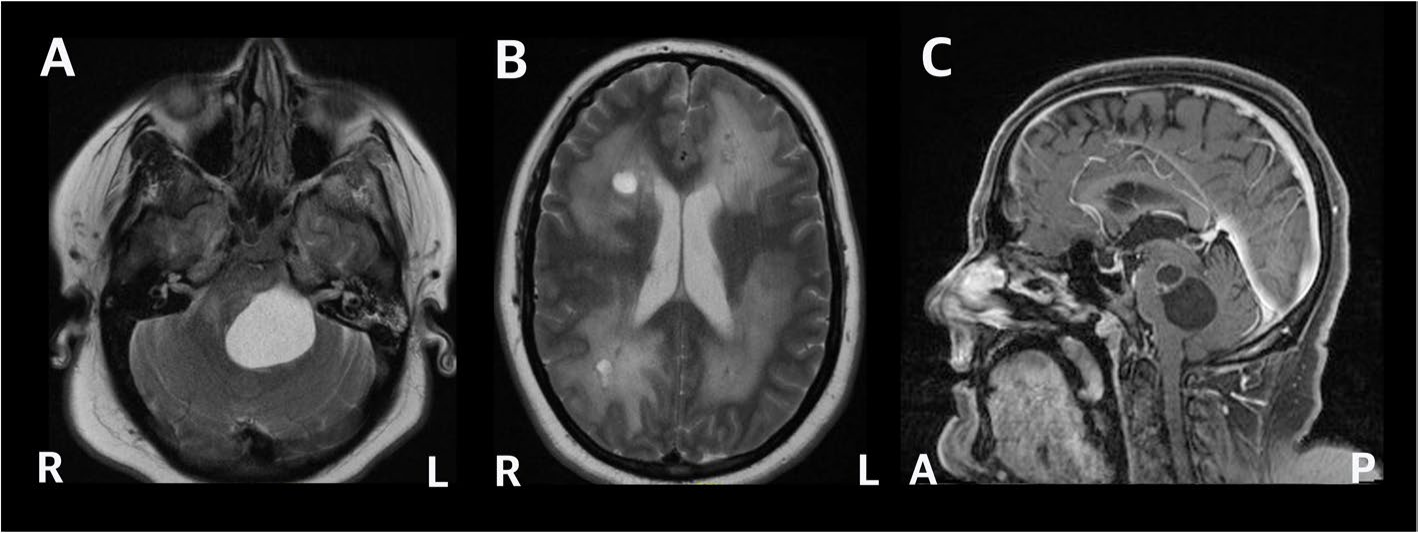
Magnetic resonance imaging (MRI) demonstrating LCC intracranial pathology. T2-weighted axial images demonstrated a cystic lesion in the posterior fossa exerting mass effect upon the brainstem (A) and diffuse T2-hyperintense signal change in the hemispheric white matter with periventricular cystic lesions (B). T1-weighted sagittal images showed the large posterior fossa cyst expanding from the dorsal pons and compressing the fourth ventricle (C)
